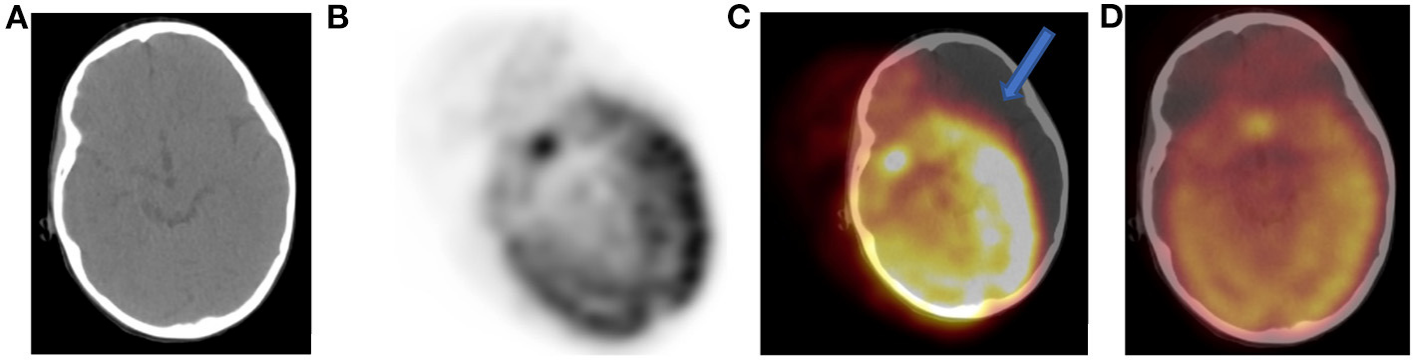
Tilt of CT (A) with gross misregistration artifact. Axial FDG PET (B) showing oblique position of brain uptake and fused PET-CT (C) images demonstrate brain FDG uptake erroneously localizing to the left outside skull (solid arrow). We used a correction software that is part of the package of our new digital PET-CT to reposition brain tissue within skull (D). The artifact results from patient motion and changing position between CT and PET acquisition.
